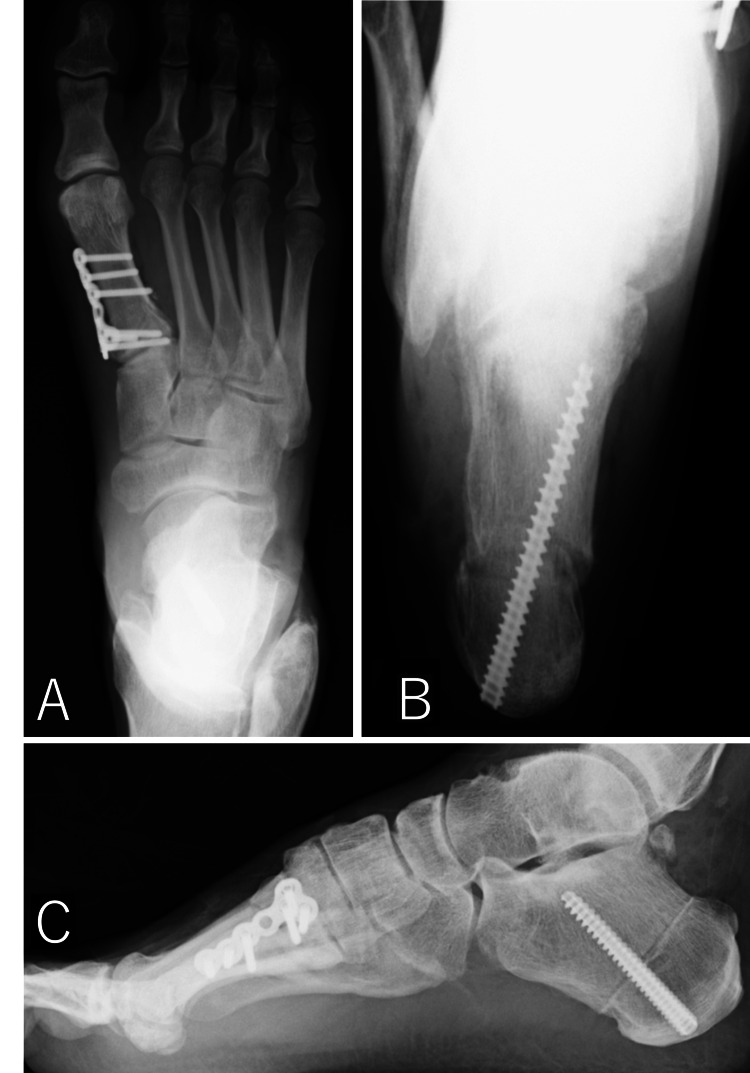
Postoperative plain radiographs (A) Dorsoplantar view. (B) Axial view of the calcaneus. (C) Lateral view.
